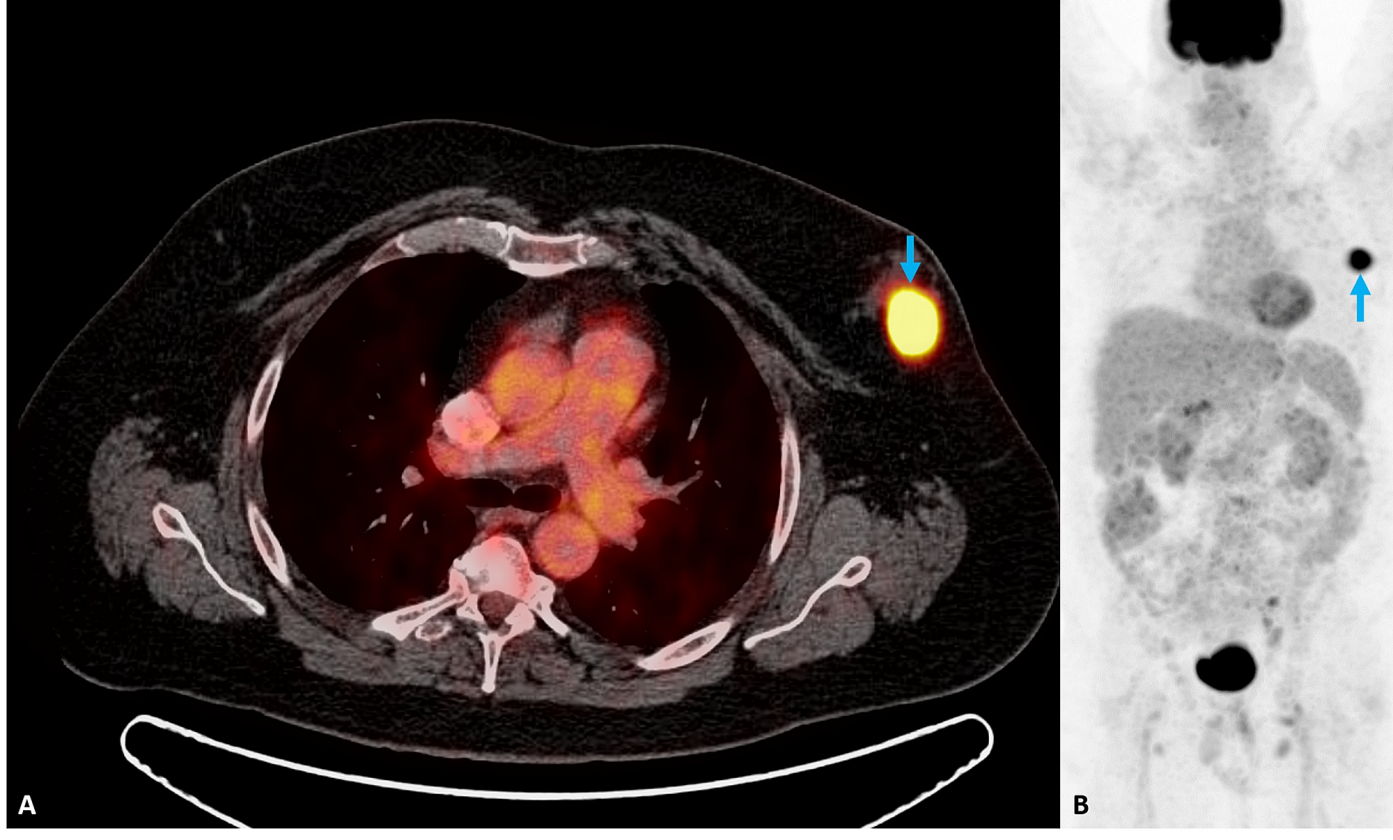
Pelvic PET/CT After initial diagnosis, further evaluation by (A) positron emission tomography/computed tomography (PET/CT) revealed a hypermetabolic area in the same location as the palpable nodule in question (blue arrow). PET imaging alone (B) revealing a hypermetabolic left breast mass (blue arrow).
